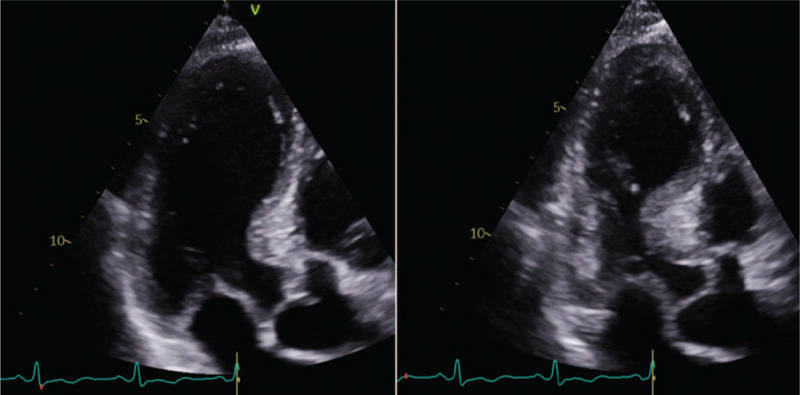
Transthoracic echocardiography images of a coronavirus disease 2019 patient with Takotsubo cardiomyopathy. Apical long-axis views. End-diastolic frame (left) and end-systolic frame (right) showing typical obstruction of the basal part of the heart and apical ballooning.
